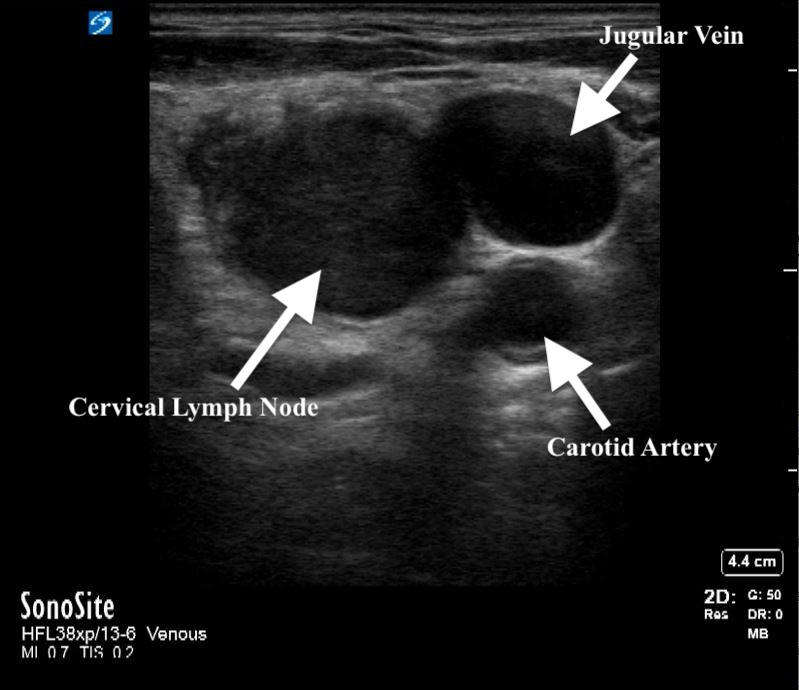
Soft tissue ultrasound image showing an enlarged right supraclavicular lymph node.
